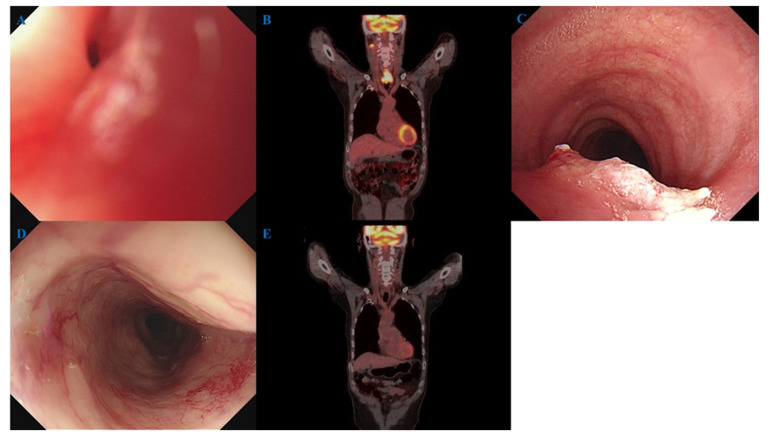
A 56-year-old male patient with cervical esophageal cancer. (A) The endoscope cannot be inserted due to a mass in the cervical esophagus. (B) FDG-PET/CT showed cervical esophageal cancer and lymph node metastasis in right neck level II. (C) Trachea invasion was observed on bronchoscopy. (D) Endoscopic findings 14 months after completion of definitive chemoradiotherapy showed no evidence of cancer. (E) FDG-PET/CT showed no evidence of cancer. FDG-PET/CT, ^18^F-fluorodeoxyglucose-positron emission tomography/computed tomography.
